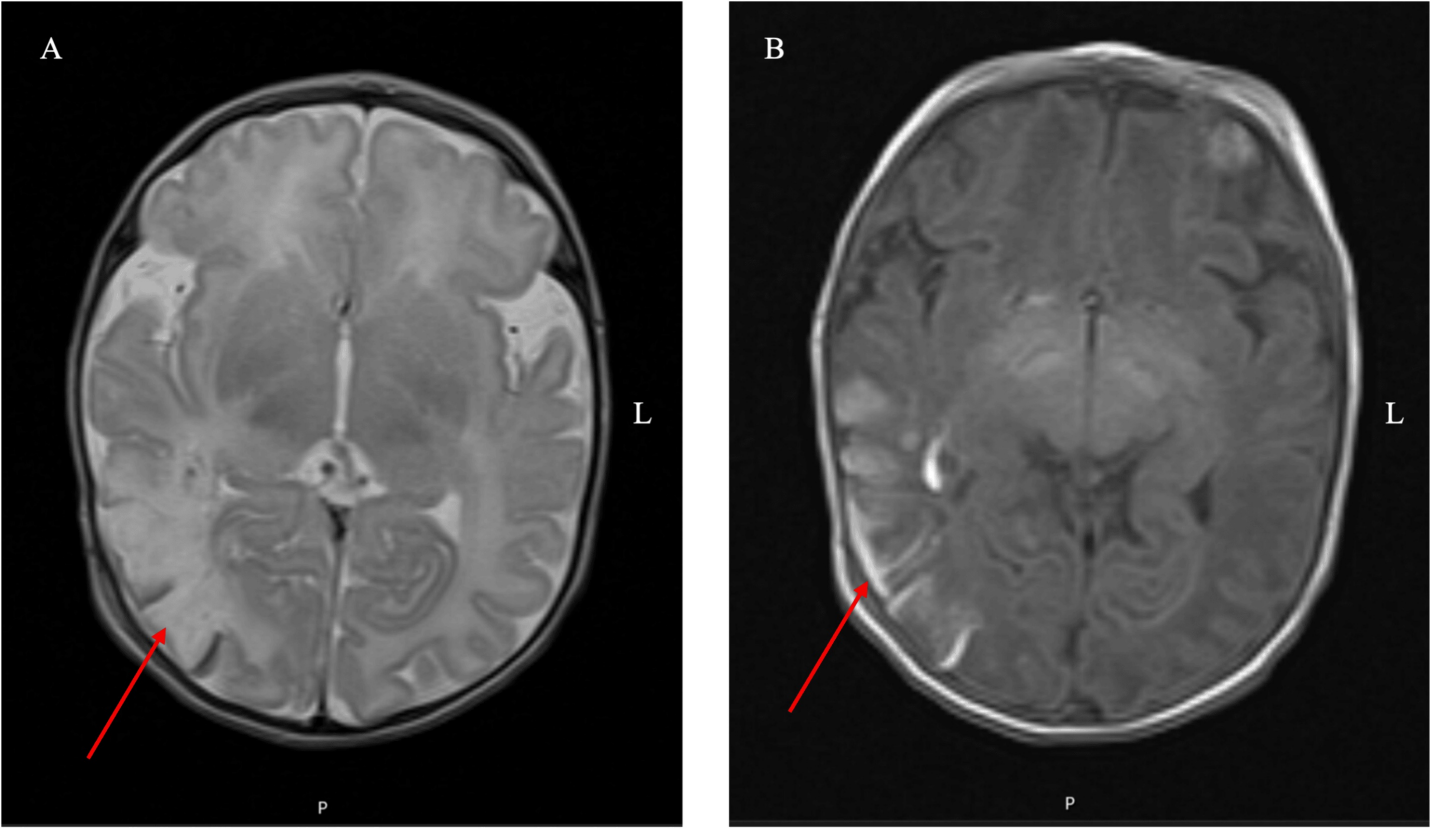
A) Brain MRI (T2-weighted imaging) showing loss of gray/white matter differentiation with abnormally high signal intensity involving the right temporal and parietal lobes in the territory of the right middle cerebral artery; B) hypersignal in the T1 sequence due to the presence of intracellular methemoglobin suggesting cortical hematic suffusion
